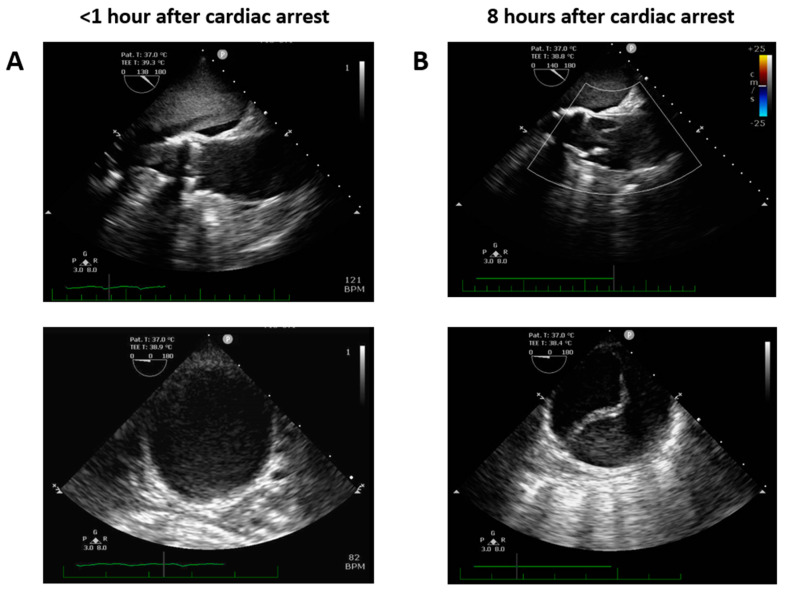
Transesophageal echocardiography focused on ascending and descending thoracic aorta performed early (A) and 8 h after cardiac arrest (B).
